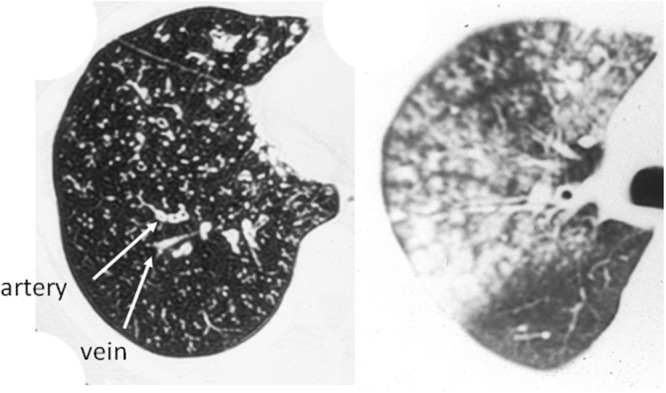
Computed tomography of centrilobular nodules predominant pattern in two patients with *M. pneumoniae* pneumonia. Reproduced with permission from Tanaka et al. (2004).
